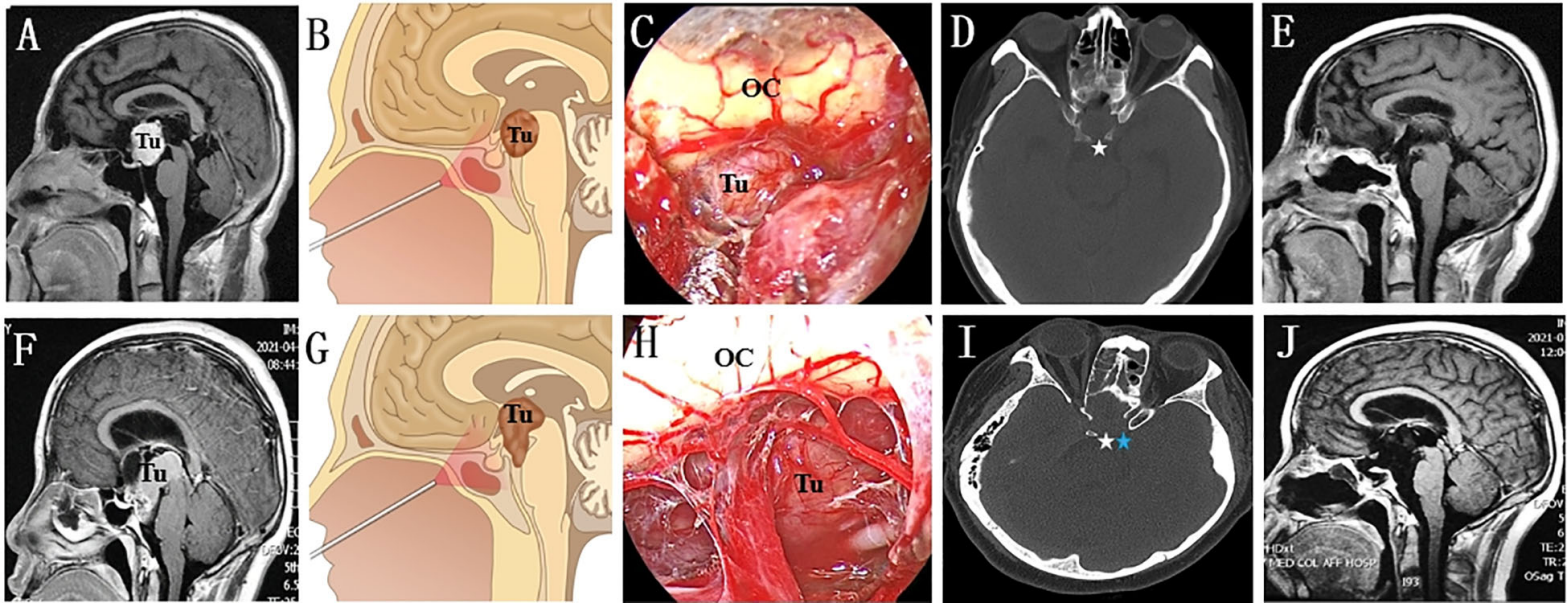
Endoscopic endonasal intradural pituitary transposition for resecting retroinfundibular lesions. (A, B) The tumour is located retroinfundibularly and extends inferiorly into the prepontine areas. (C) The infrachiasmatic space was narrow, and the tumour was blocked by the PG and DS. (D) The DS was individually drilled out (white arrow) and confirmed by a postoperative thin-layer CT scan. (E) Follow-up MR image showing that the tumour was radically resected. (F, G) This retroinfundibular tumour was very large and occupied both the prepontine and interpeduncular cisterns. (H) Except for the DS, the surgical corridor was effectively enlarged by removing the left PCP. (I) The left DS (white arrow) and PCP (blue arrow) disappeared on the postoperative thin-layer CT scan. (J) Follow-up MR image showing no residual tumour or occurrence. Tu, tumour; OC, optic chiasma.
